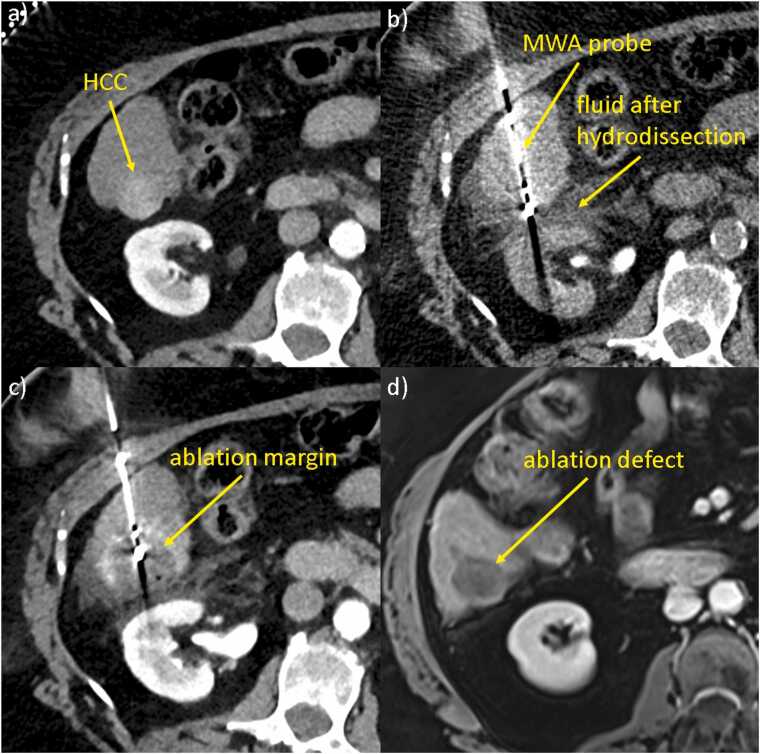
Case example of a successful MWA of an HCC in subcapsular location in segment 6. a) initial planning CT in arterial phase showing the hyperarterialized lesion. b) the MWA probe is precisely placed. A hydrodissection was performed to increase the distance to the right kidney. c) Immediately after the ablation a control CT scan is performed with the probe still in place, showing a complete ablation with a sufficient safety margin and without any complications. d) MRI control 4 weeks after showing the ablation defect without any evidence of rest tumor.
